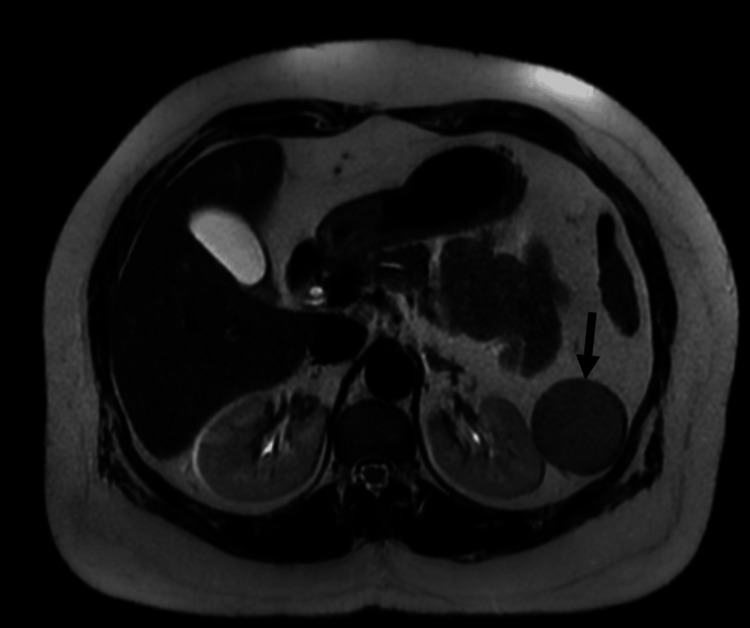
Abdominal MRI (axial single-shot fast spin-echo (SSFSE) image) demonstrating a well-circumscribed lesion (arrow) in the splenic fossa, showing homogeneous signal intensity similar to splenic tissue, without internal heterogeneity or aggressive features, favoring splenosis
